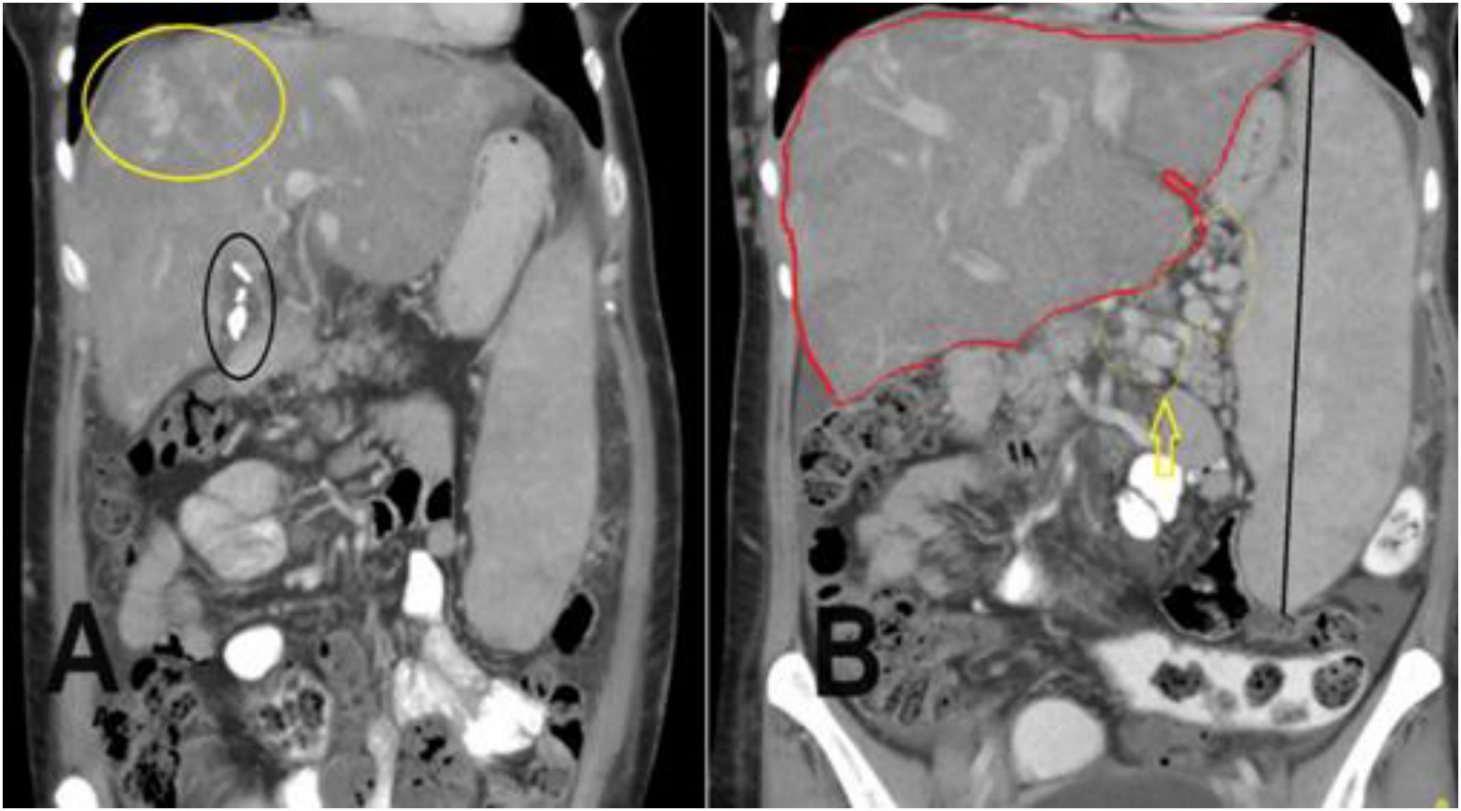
A coronal section of the portal venous phase of the computed tomography (CT) abdomen for the previously mentioned patient as in Figure 1 demonstrates (A) incidental finding of cholecystectomy clips and fluid in the gallbladder fossa indicating recent cholecystectomy. (B) Hepatomegaly and a huge spleen are consequences of Budd-Chiari syndrome (BCS), splenic varices (yellow arrow), and multiple collateral veins in the abdominal wall.
